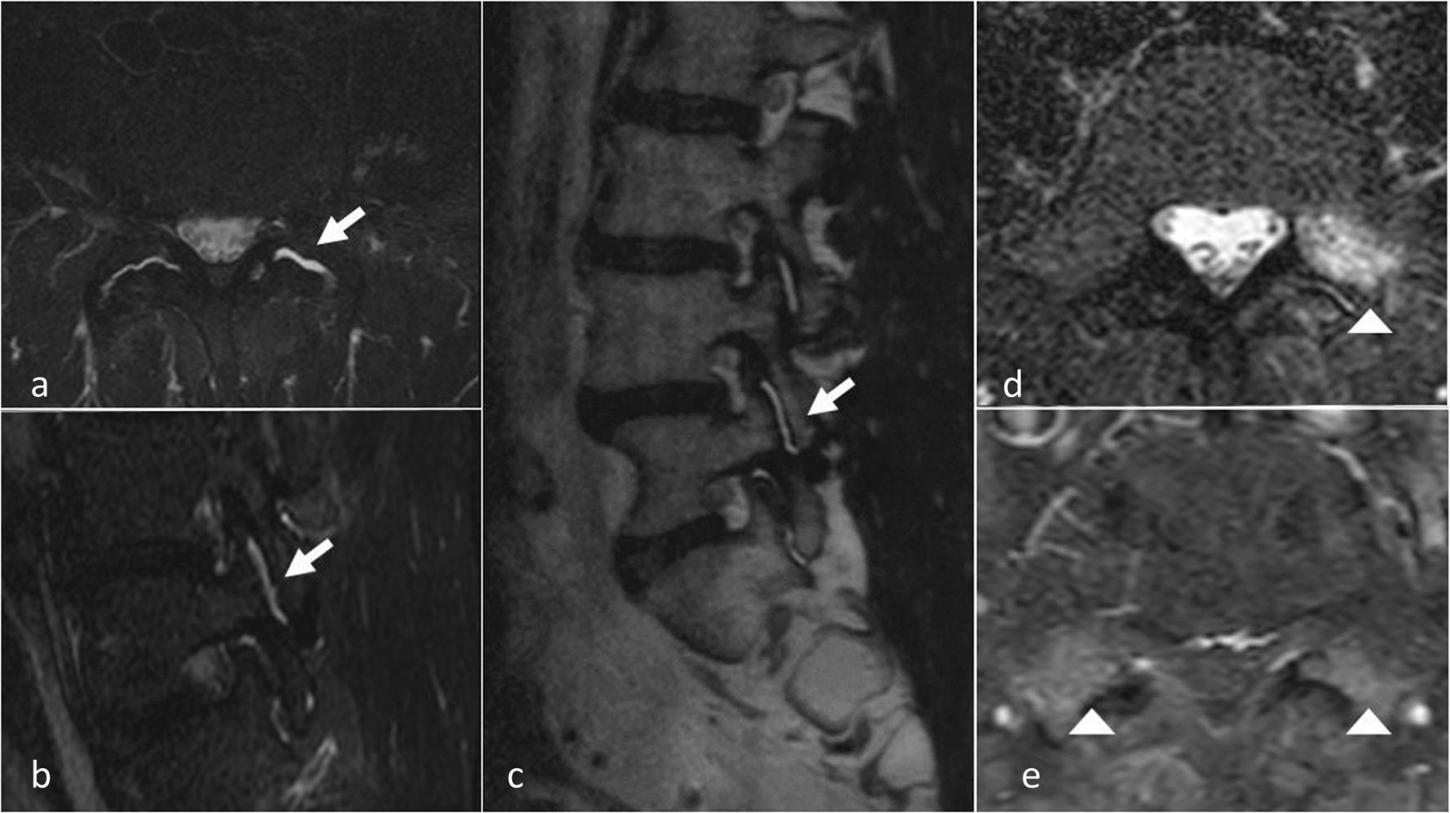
MRI imaging of facet joints. Active synovial inflammation and intra articular edema: axial and sagittal T2 STIR views (a, b) and T2 sagittal view (c). T2 STIR and T1 gado axial views (d, e): articular process bone edema
